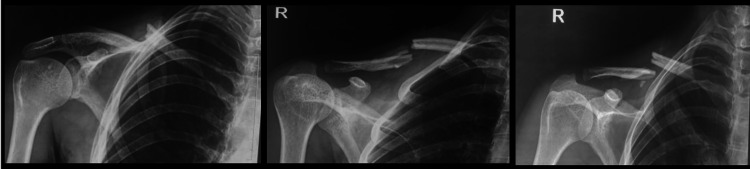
Displaced clavicle fracture - AP, cephalic, and caudal X-ray views
